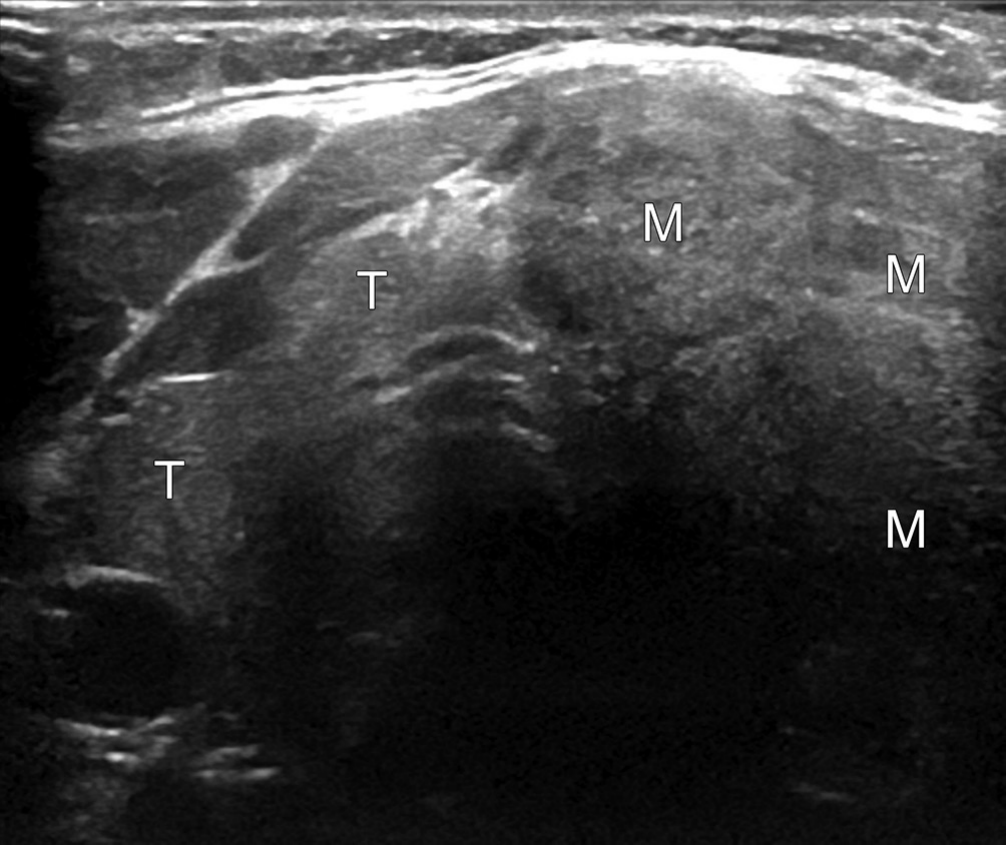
Transverse sonogram of the thyroid. Normal right lobe and isthmus (T). There is a large mixed echogenicity mass partly delineated in the thyroid left lobe (M).
